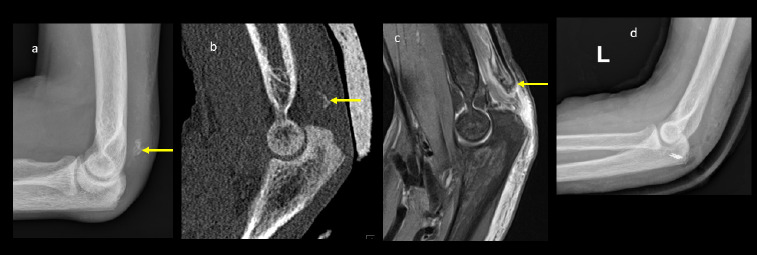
Left elbow a) lateral plain radiograph b) CT sagittal reformats c) Sagittal PD FAT SAT MRI showing avulsion fracture of olecranon (triceps attachment site) with associated posterior soft tissue swelling. The left sided avulsion (yellow arrow) fracture is more displaced compared to the right. Ultrasound scan of the left elbow was not performed due to presence of back slab on the left. d) Post operative plain lateral radiograph of left elbow showing screw fixation of olecranon avulsion fracture.
